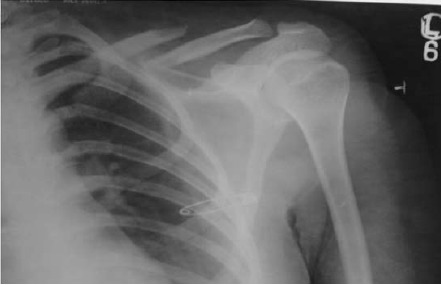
Fig. 2: Post- operative radiograph of left shoulder F2:
